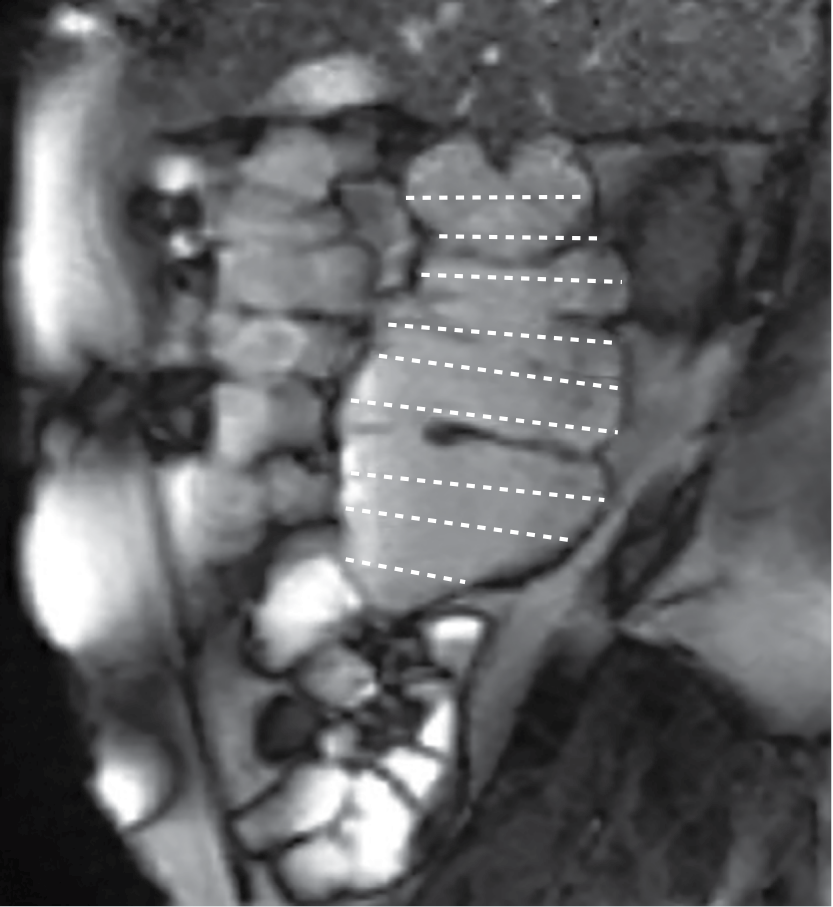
MRI assessment of colonic wall movement. MRI enables the measurement of various characteristics of the gastrointestinal tract, such as organ volumes, transit rate and motility. In this MRI of a sagittal section of the ascending colon, the horizontal lines define the colonic lumen. During cine MRI, changes in the lengths of the lines provide a measure of transverse wall velocity, which enables the calculation of a motility index (the percentage of data points at which the wall velocity is >0.5 mm/s).
